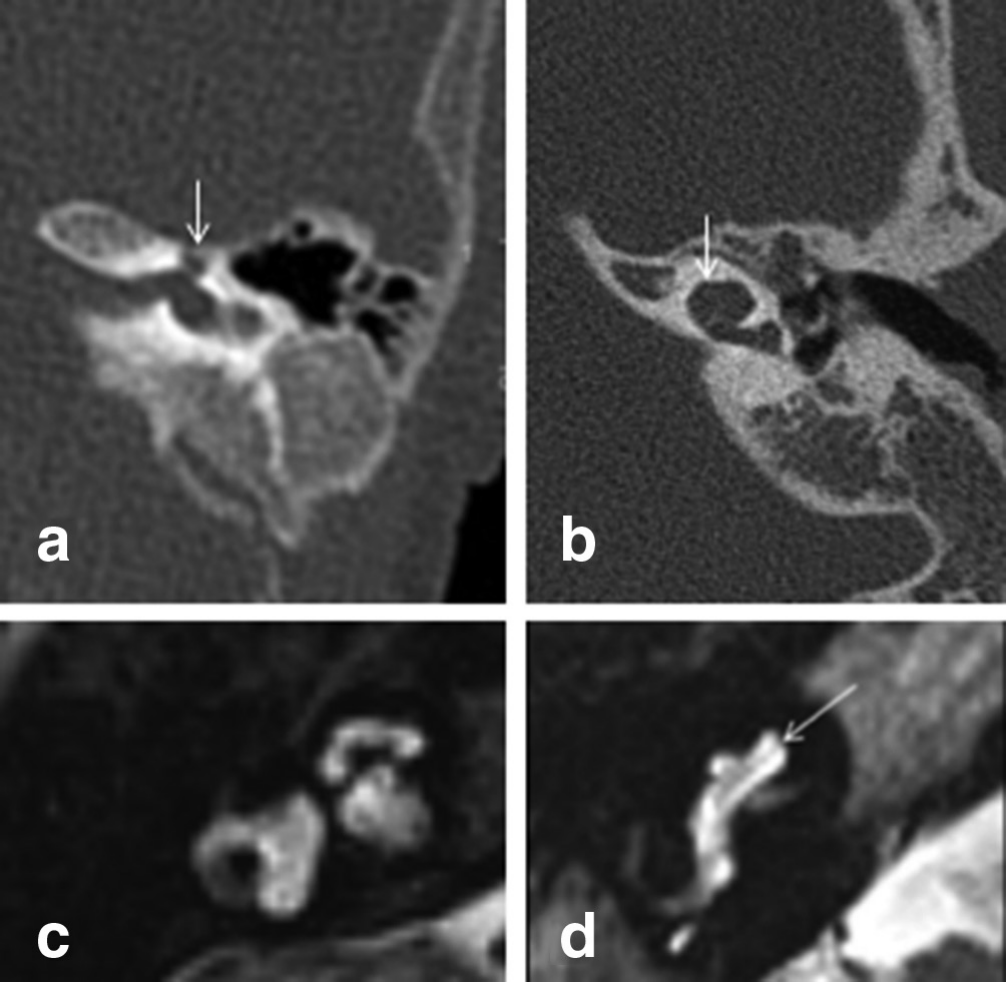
CT appearances of cochlear hypoplasia Type 1 (arrows in A) and Type 2 (arrow in B). Two different MRI appearances of cochlear hypoplasia Type 3 (C) and 4 (D) with relatively preserved internal structure and normal sized basal turn noted only in Type 4 (arrow in D).
